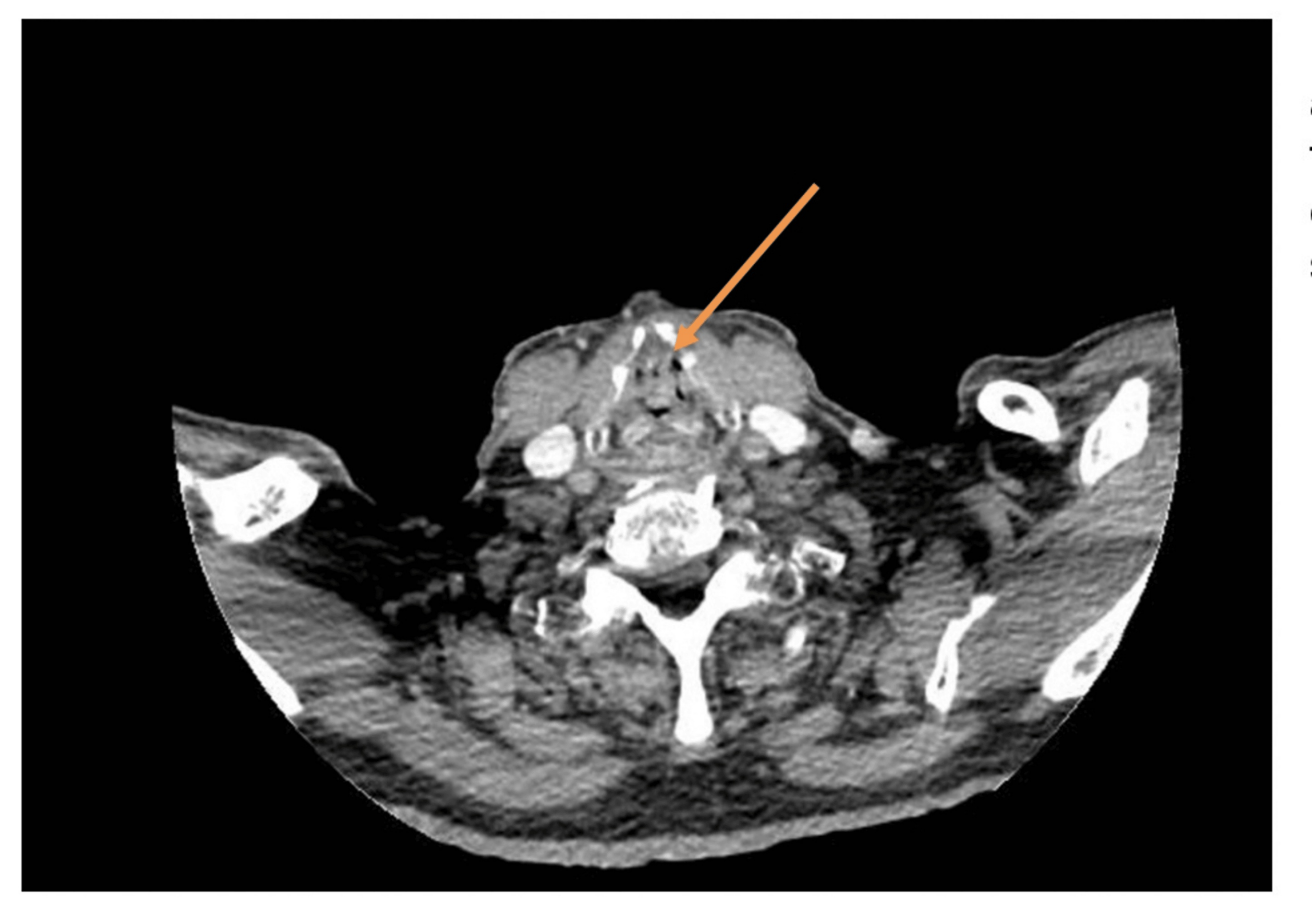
CT scan taken on admission showing soft tissue filling defect in the glottic and supraglottic airway. The arrow denotes the soft tissue filling defect in the glottic and supraglottic airway.
